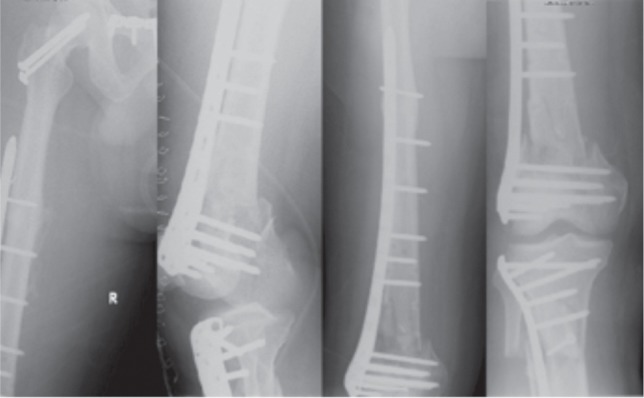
Radiographs post definitive fixation following polytrauma.
